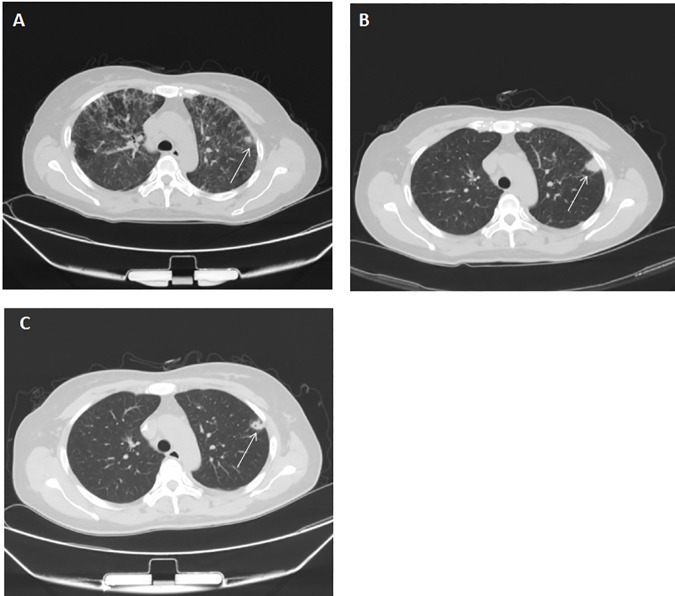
A representative case of clinical deduction of pulmonary cryptococcosis. A 47-year-old woman presented to hospital with fever, cough and shortness of breath. She was confirmed of HIV infection with a CD4 count of 60 cells/μL. The serum cryptococcal antigen titer was 1:16. Lumbar puncture was performed and there was no evidence of cryptococcal meningitis. A chest CT scan showed diffuse ground-glass opacities (DGO). Also on the Chest CT, there was a small nodule (A, arrow). She was given empirical treatment with co-trimoxazole (1440 mg, every eight hours) for pneumocystis pneumonia, and methylprednisolone as an adjunctive therapy. A chest CT scan after 3 weeks showed nearly complete resolution of the DGO; however, the nodular lesion progressed(B, arrow). The patient then received secondary prophylaxis for pneumocystis pneumonia with co-trimoxazole(960 mg/d) and treated with fluconazole (400mg/d). A follow-up CT scan after seventeen days of fluconazole treatment showed partial resolution of the nodule (C, arrow). Taken together, the nodule lesion was attributed to pulmonary cryptpcoccosis, while the DGO were related to pneumocystis pneumonia.
